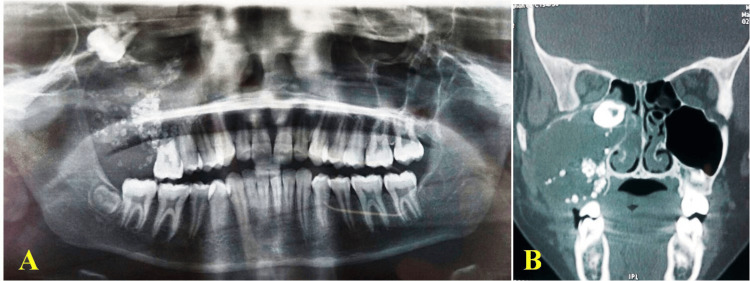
(A) Orthopantamogram with mixed radiolucency and radioopacity; (B) cone-beam computed tomography with obliteration of right maxillary sinus, impacted molar, and numerous radiopaque masses
